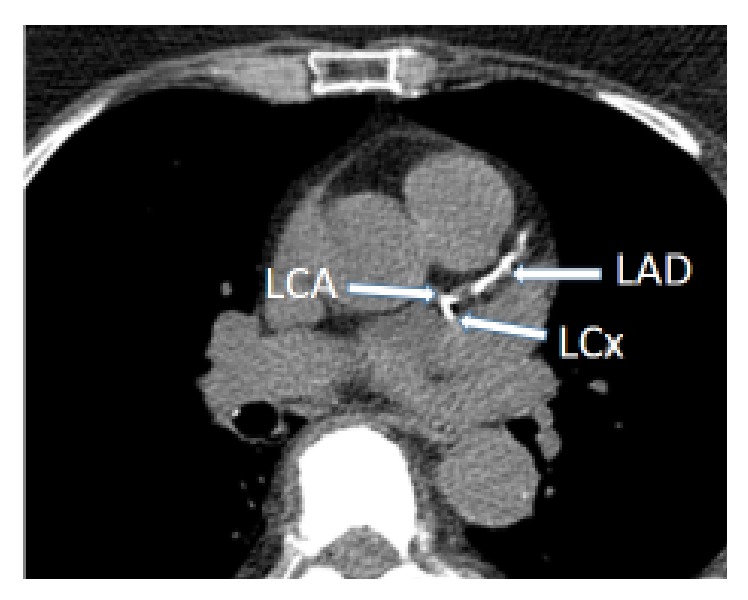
CT scan. Calcifications are seen in the bifurcation of the left coronary artery (LCA), the proximal part of left circumflex artery (LCx), and the left anterior descending artery (LAD). Noncalcified parts are visible both proximal and more distal in LAD, seen as low-attenuating areas between the calcified parts.
